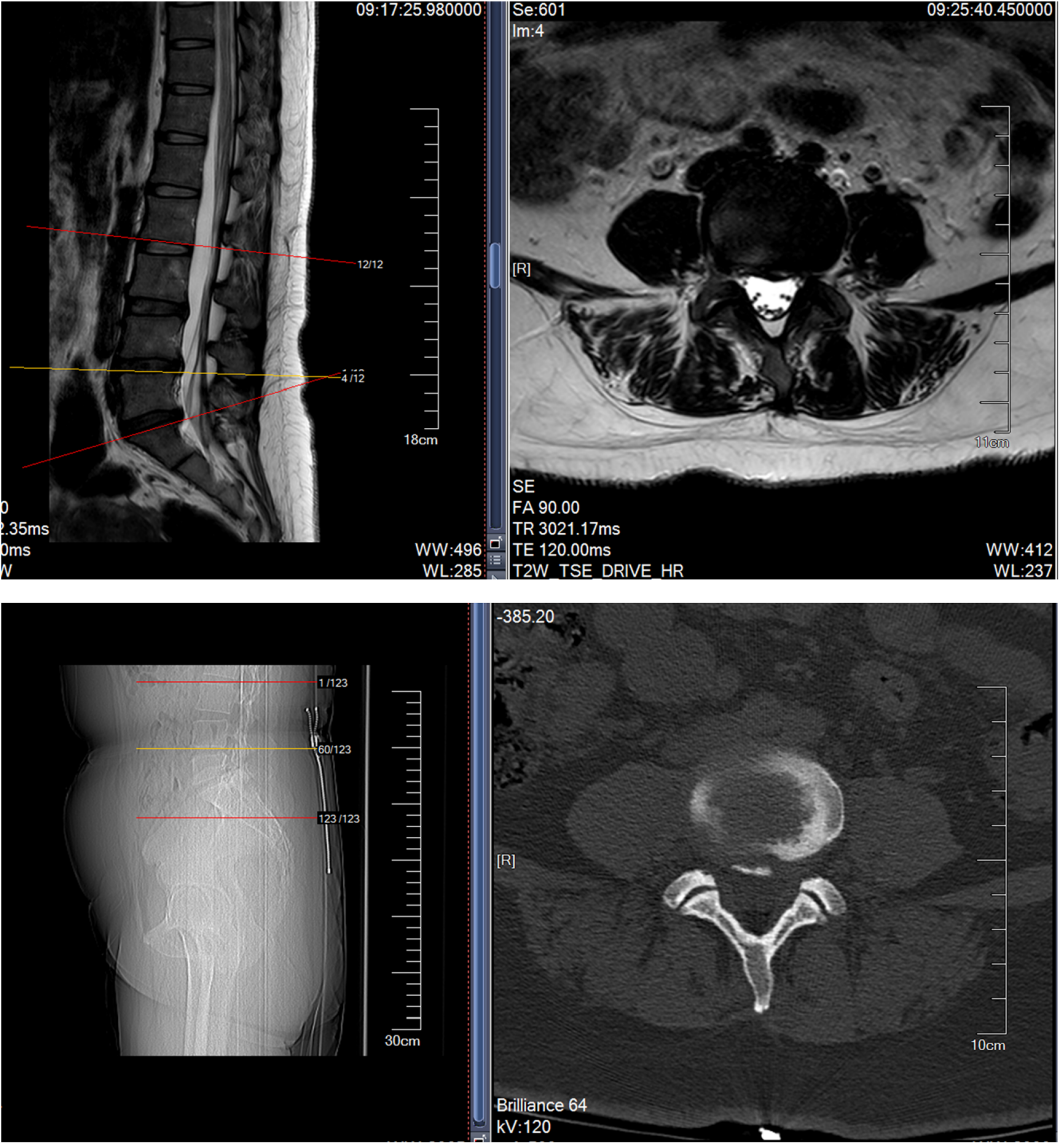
Shows that MRI indicates herniation of the L3/4, L4/5 and L5/S1 intervertebral discs, while CT suggests calcification of the posterior ligament.
